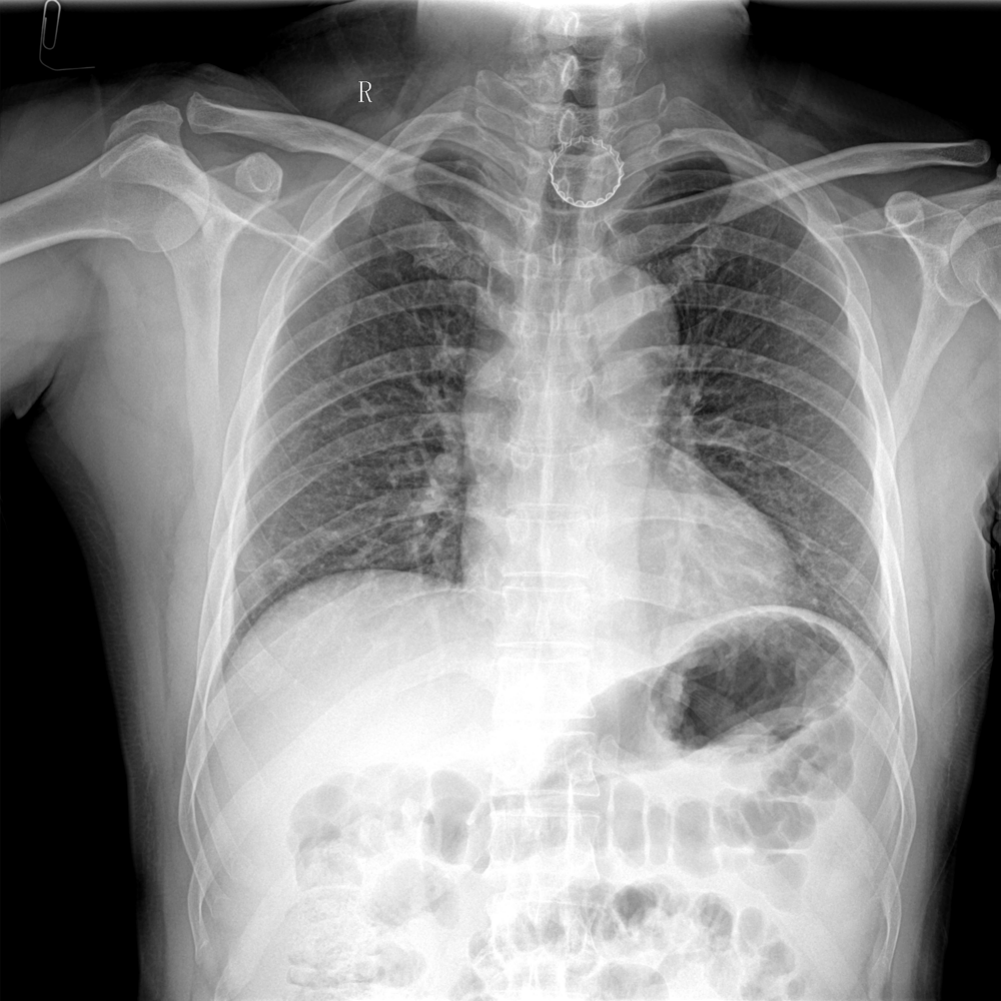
Digital radiography image revealed circular radiopacity at T1-T2 level (arrow).
